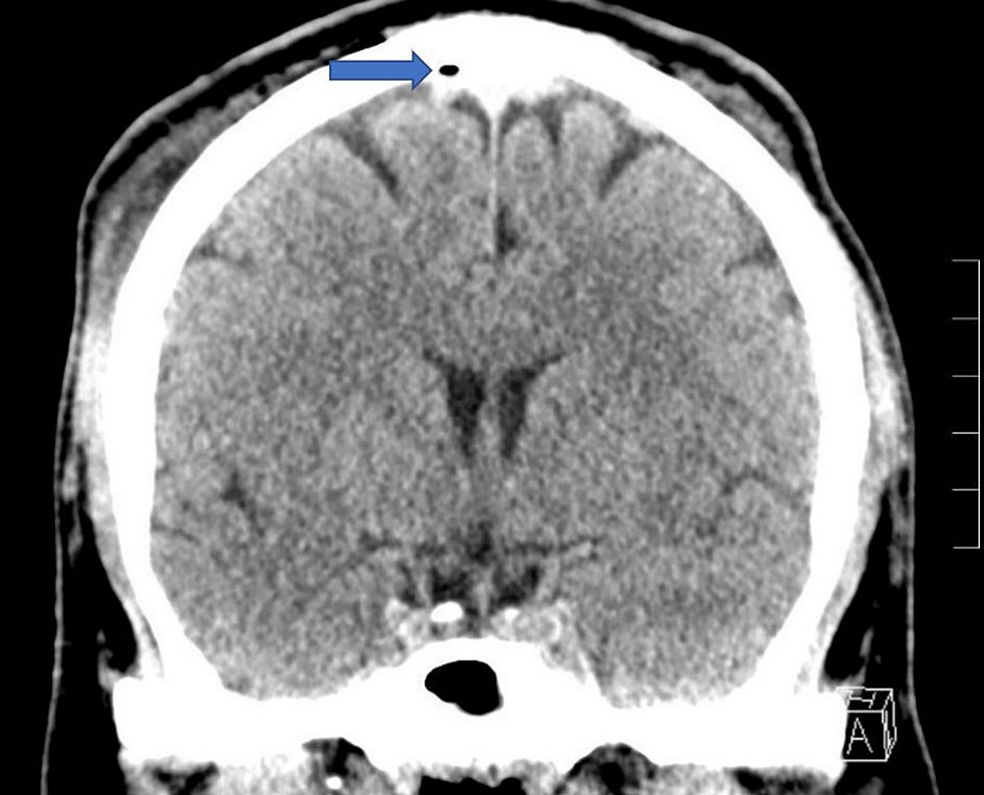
Coronal slice of a noncontrast CT demonstrating a small focus of air within the sagittal sinus without skull fracture as well as a slight displacement of the superior sagittal sinus from the adjacent skull, concerning for a possible small epidural hematoma measuring 4 mm in thickness without mass effect. CT: computed tomography
